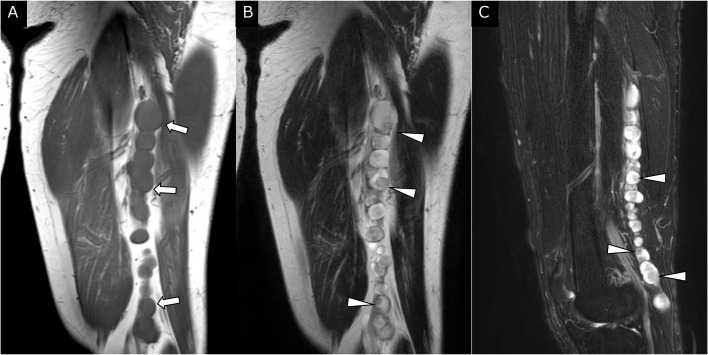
MRI of the left thigh. (A) Coronal T1WI showed a bead-like multinodular structure (arrows) with uniform signal intensity compared with skeletal muscle. (B) Coronal T2WI and (C) sagittal fat-suppressed T2WI showed multiple well-defined bead-like structures with localization corresponding to the sciatic nerve pathway. These nodules showed heterogeneous high signal intensity and had a low-intensity rim. Eccentric intratumoral areas of relatively low signal intensity (arrowheads) were also observed.
